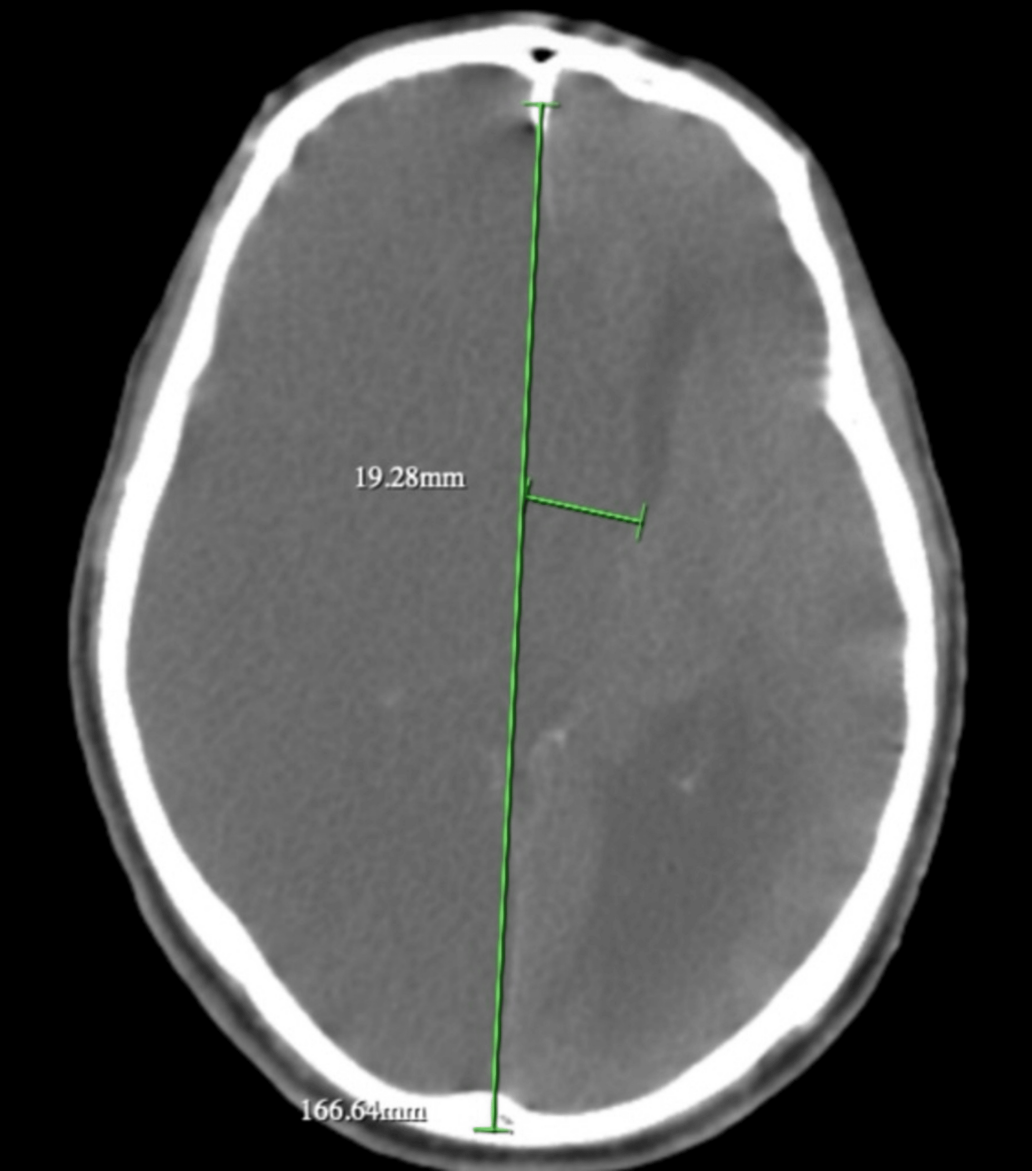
Head CT without contrast displaying 19.28 mm midline shift in the left hemisphere CT: computed tomography
